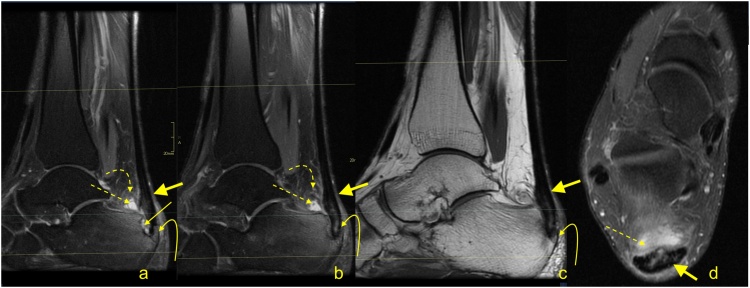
Advanced stage of Haglund's syndrome. MRI revealed synovitis in the retrocalcaneal bursa (dashed arrow) with edema in Kager's fat pad (curved arrow). In the calcaneus intraosseous cyst (straight arrow) with extensive bone marrow edema is visible. The enthesophyte with bone marrow edema is visible in the superficial part of the tendon's insertion (curved arrow). The Achilles tendon in the distal 1/3 shows thickening and alteration in signal (thick arrow). A and b- PD- weighted with fat suppression, c- PD- weighted, d- PD-weighted with fat suppression.
